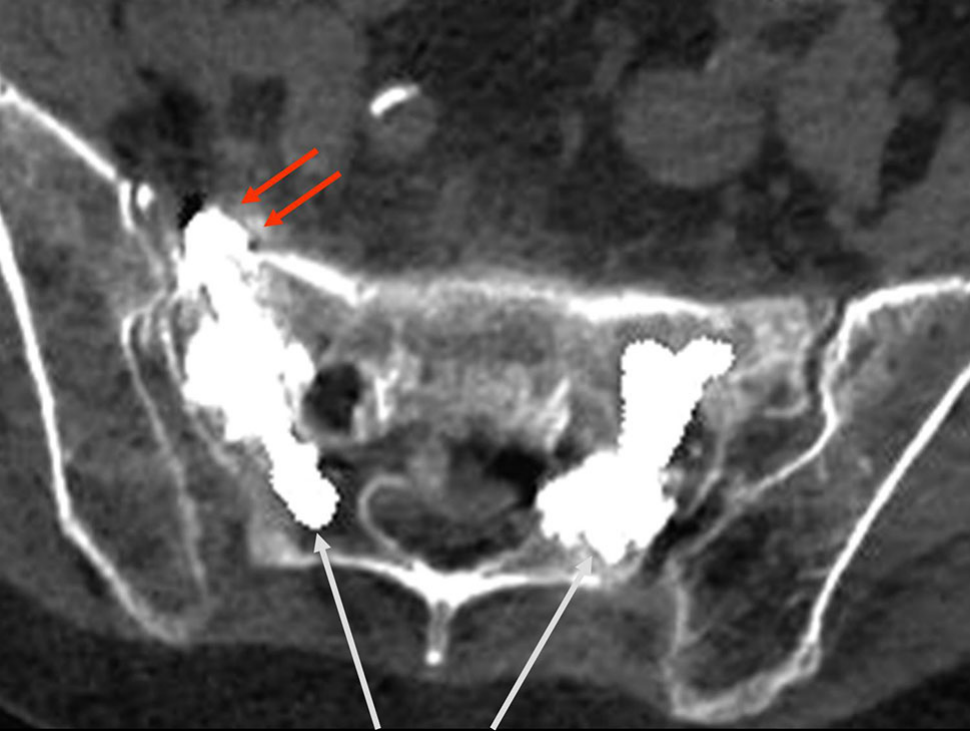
Axial CT image with a layer thickness of 2 mm shows a cement leakage from the fracture zone beyond the cortical boundary of the visceral surface of the sacrum on the* right side* (marked with* red arrows*). On both sides, a Denis type 1 fracture zone was augmented with cement. The approaches via the short axis are marked with* grey arrows* (colour figure online)
